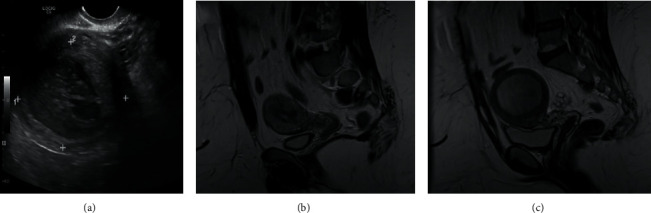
(a) 2D scan read as fibroid with central necrosis. (b, c) Communicating and noncommunicating horns on MRI.
